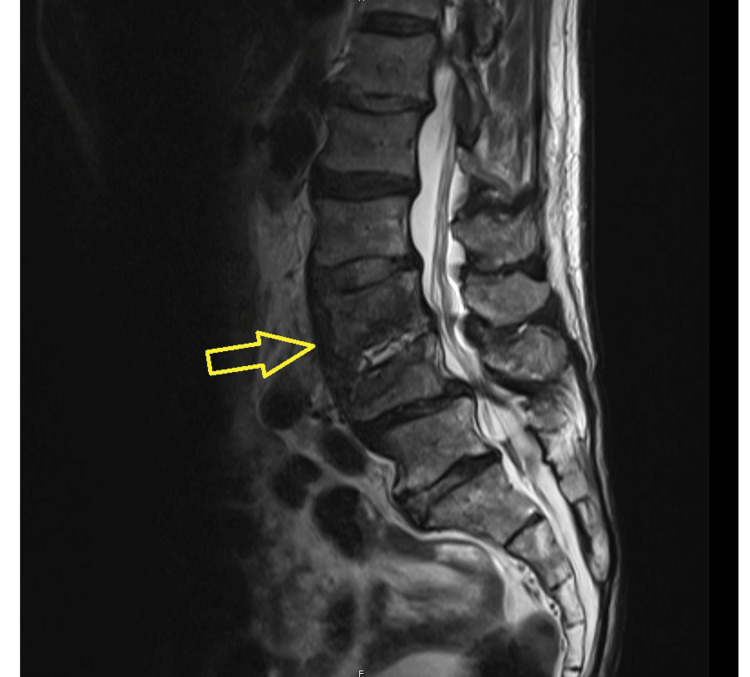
Sagittal T2-weighted MRI of the lumbar spine Arrow highlights diffuse enhancement of L3 and L4 which extends into the pedicles. There is also edema in the pre vertebral soft tissues at L3 and L4. Additional findings on the MRI include multilevel degenerative changes and ligamentum flavum hypertrophy present.
